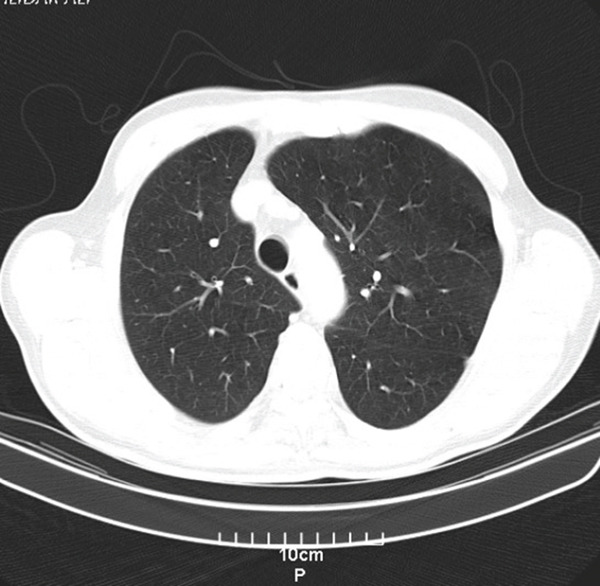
Chest computed topography (CT scan), hyperinflation of the left lung, and shifting the mediastinum to the right.
